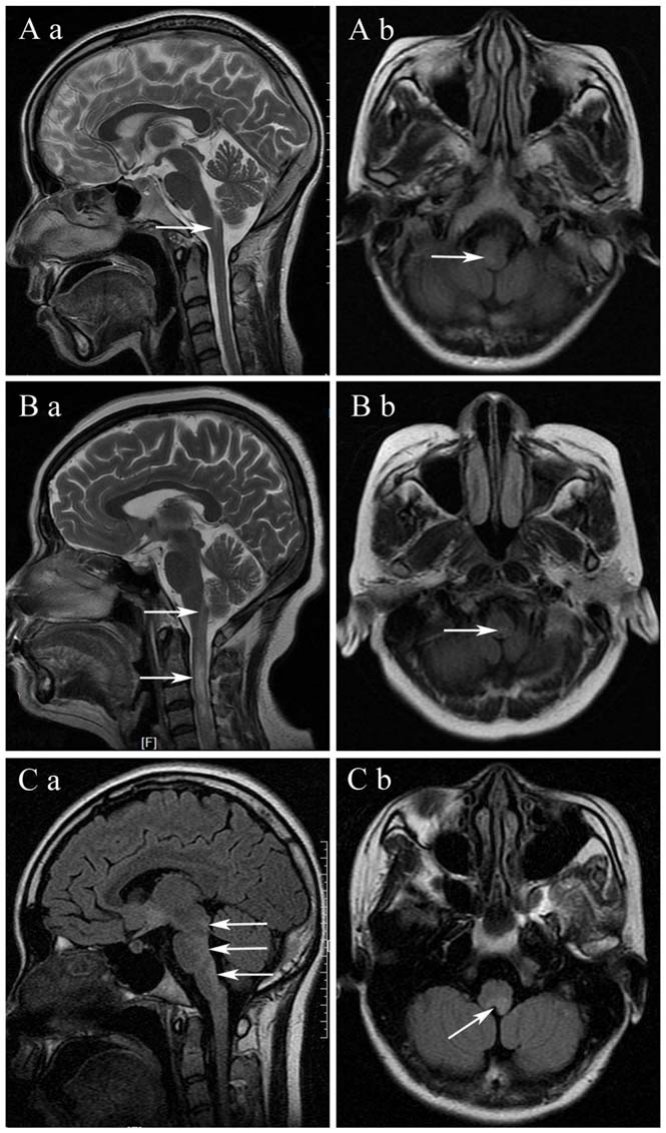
Brain stem lesions (arrows) on MRI of neuromyelitis optica (NMO). (A) MRI showing medulla lesion located in the dorsal part with poorly defined shape. (B) MRI showing linear medullospinal lesions. (C) MRI showing distribution of NMO-characteristic brain lesions (dorsal brain stem) corresponding to sites of high NMO-IgG expression.
